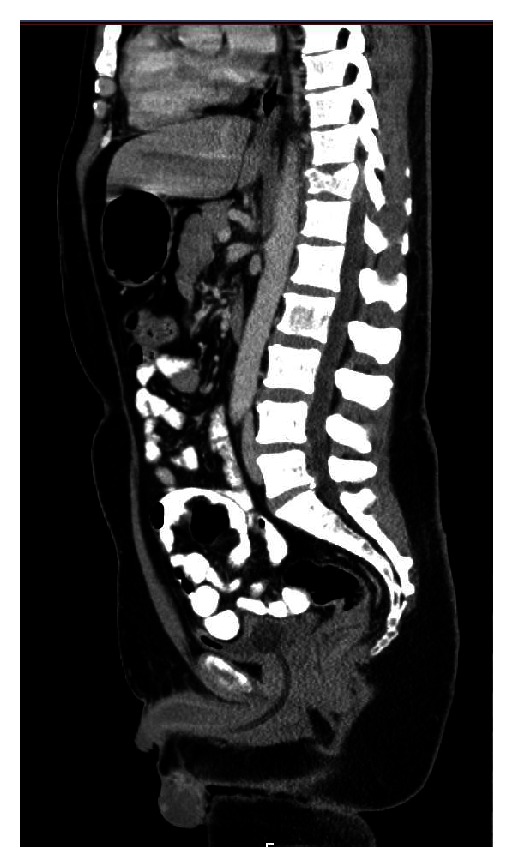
CT scan showing diffuse lytic lesions throughout the axial skeleton.
